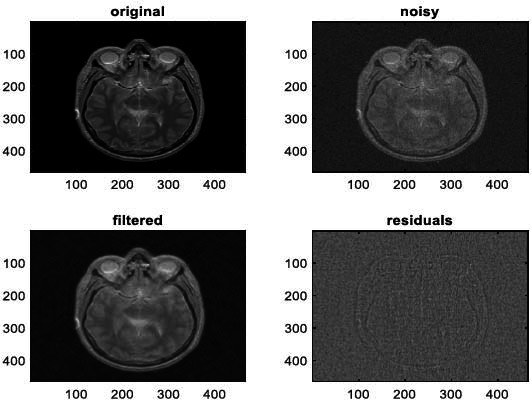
The seventh example of MRI denoising by applying the proposed image denoising technique.
